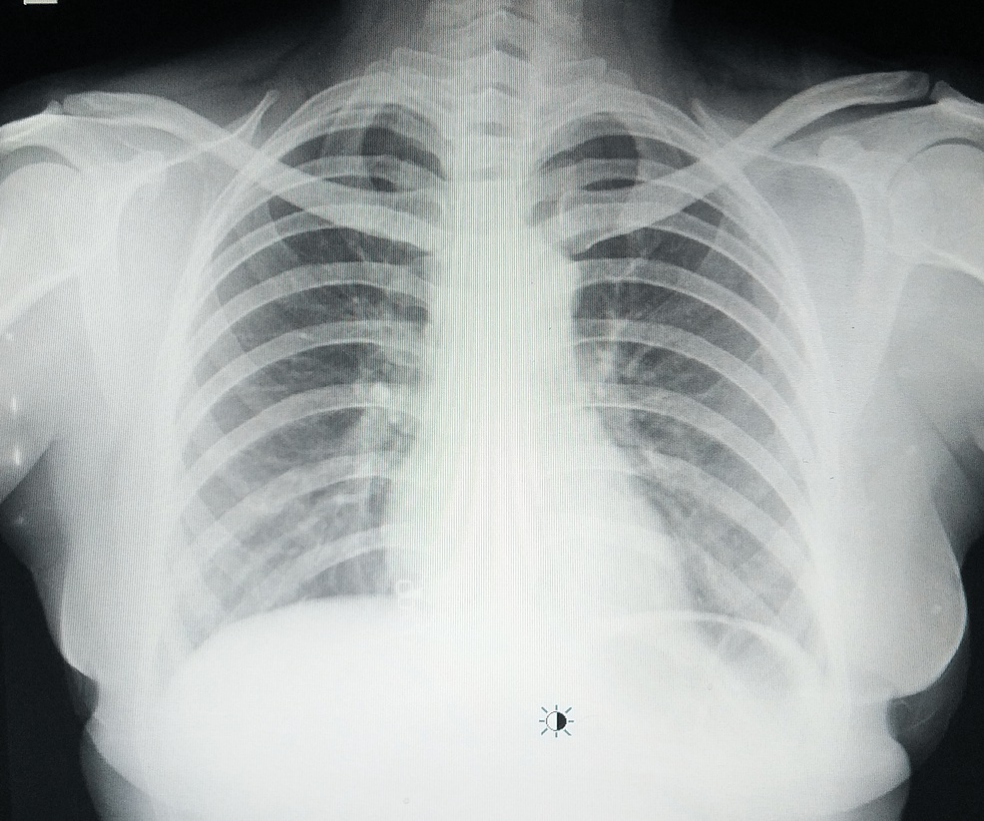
A normal plain chest radiograph antero-posterior view
